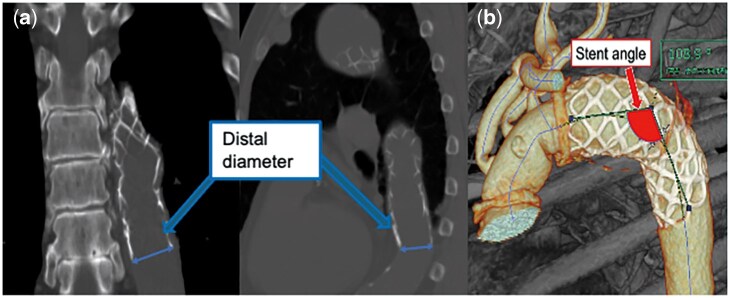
Measurement of stent angle and distal diameter. (a) Method for measuring the stent angle, i.e. the angle formed between the proximal and distal ends of the stent. (b) Method for measuring the distal diameter of the stent. The distal diameter is the diameter at the distal point of the stent and is calculated as the average of measurements obtained from computed tomography coronal and sagittal views.
